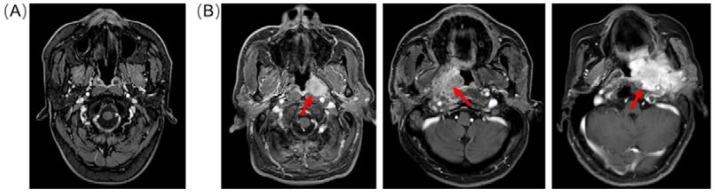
Example of contrast-enhanced T1-weighted MRIs. (A) The image from normal control without NPC cancer; (B) the images from patients with varying sizes of NPC. The arrows indicate the location of the NPC tumor.
